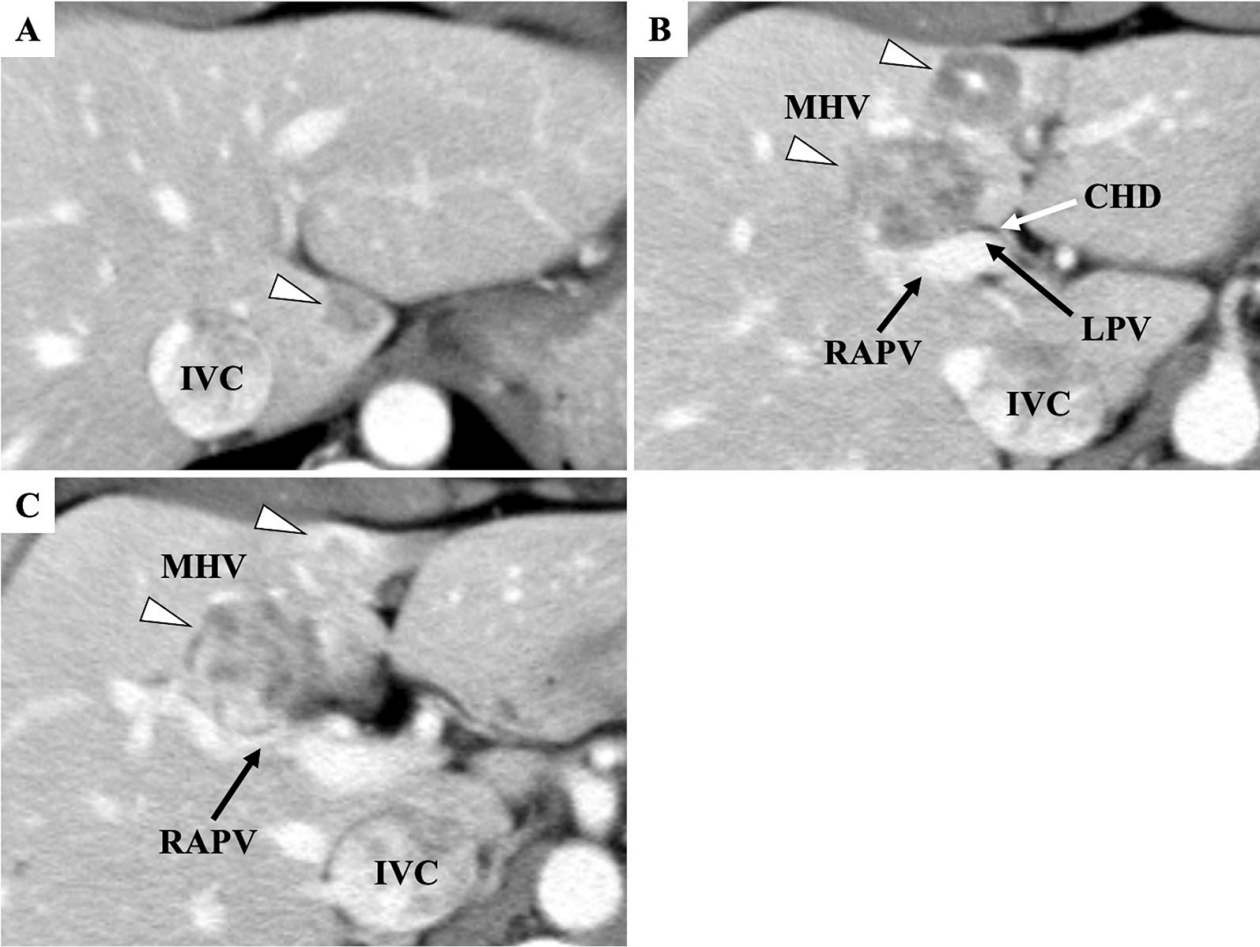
Computed tomography shows hypervascular tumors of the liver in segment 1 (A) and segment 4 (B). IVC, inferior vena cava; MHV, middle hepatic vein; LPV, left portal vein; RAPV, right anterior portal vein; CHD, common hepatic duct
